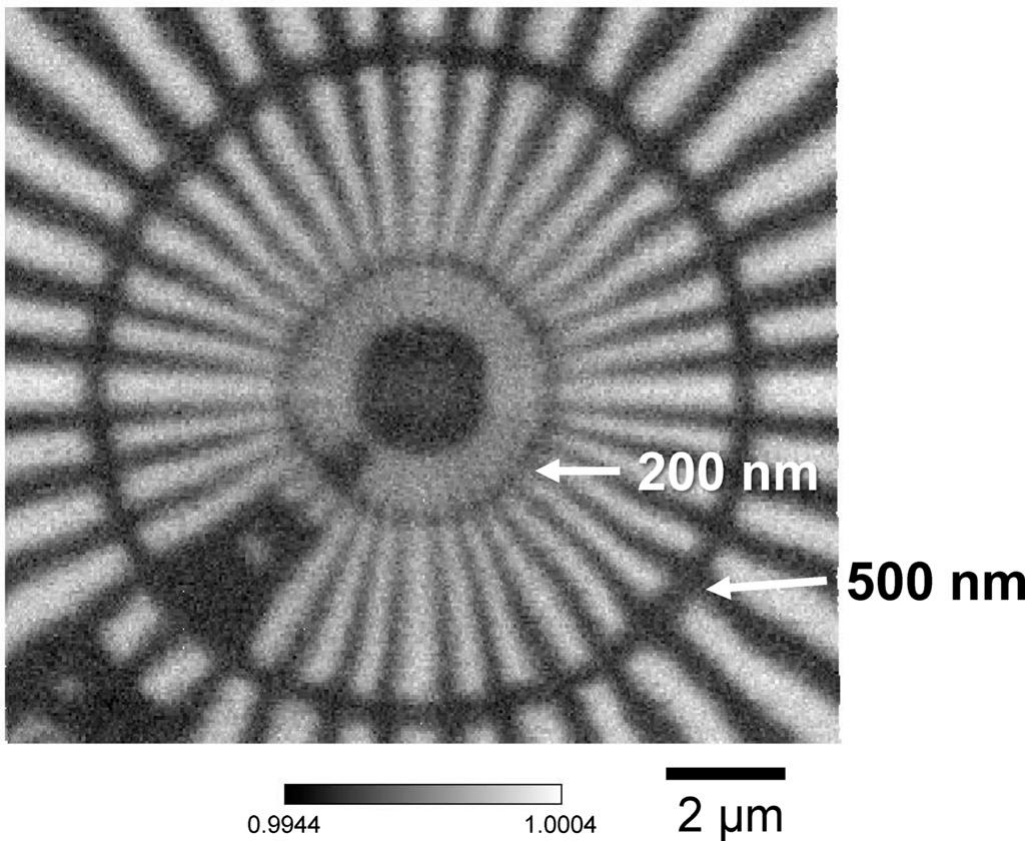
The scanning transmission image of a tantalum Siemens star chart. We used 100 keV X-rays with a focusing-beam size of 0.25 µm (V) × 0.26 µm (H). The structure of 200 nm line and space was clearly observed.
